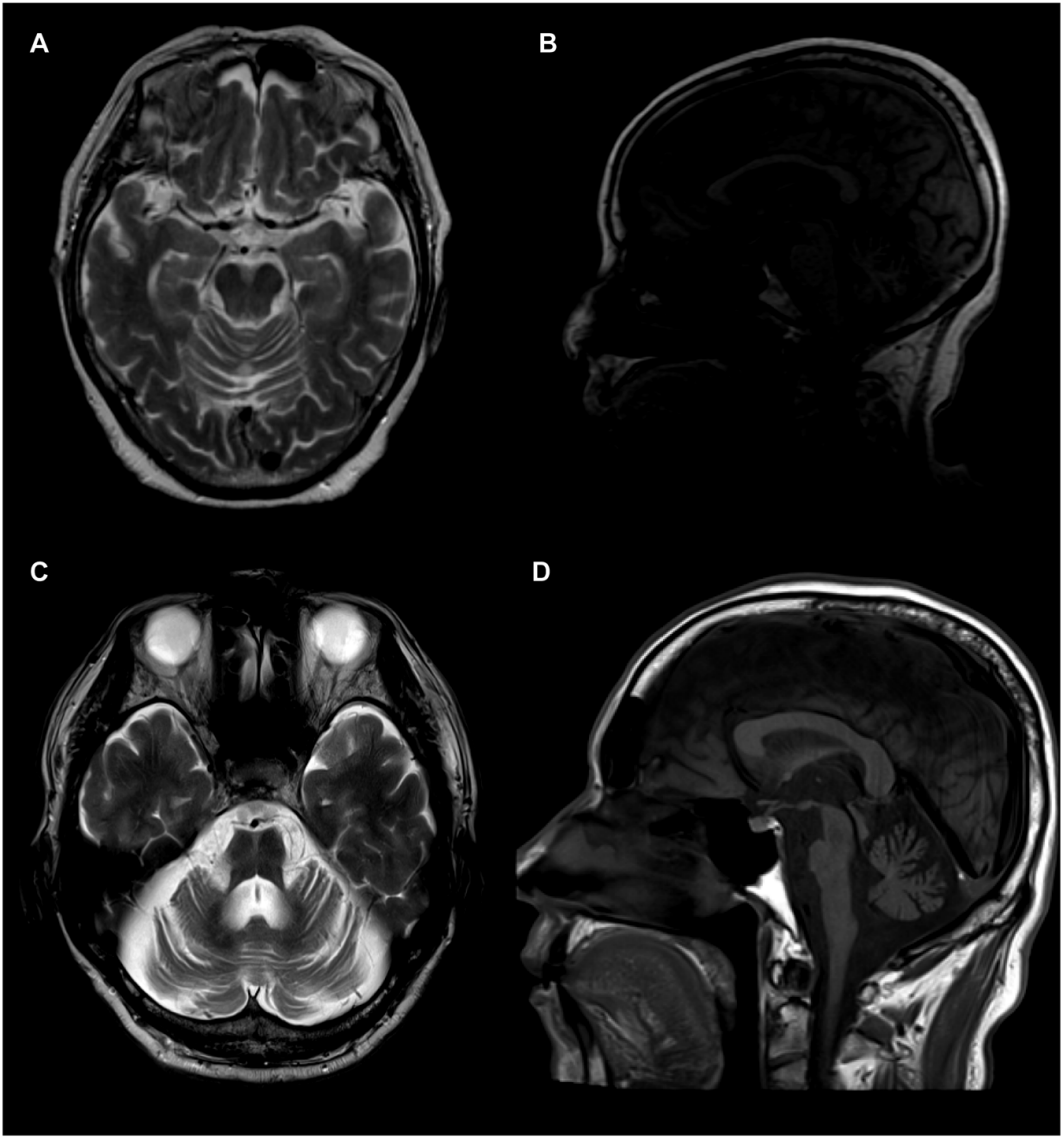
Brain MRI of the patients with MSA or undiagnosed ataxia with expanded GAA repeats. Axial T2-weighted image (A) and sagittal T1-weighted image (B) of the patient with undiagnosed ataxia who had (GAA)_312_. MRI was performed at the age of 84, twelve years after the symptom onset. Atrophic changes in the upper part of the cerebellar hemisphere and the vermis were observed. The brainstem was not atrophic. Axial T2-weighted image (C) and sagittal T1-weighted image (D) of the patient with MSA who had (GAA)_269_ seven years after the symptom onset. Atrophic changes in the cerebellum, middle cerebellar peduncles, and basis pontis were observed. The hot cross bun sign was observed in (C).
